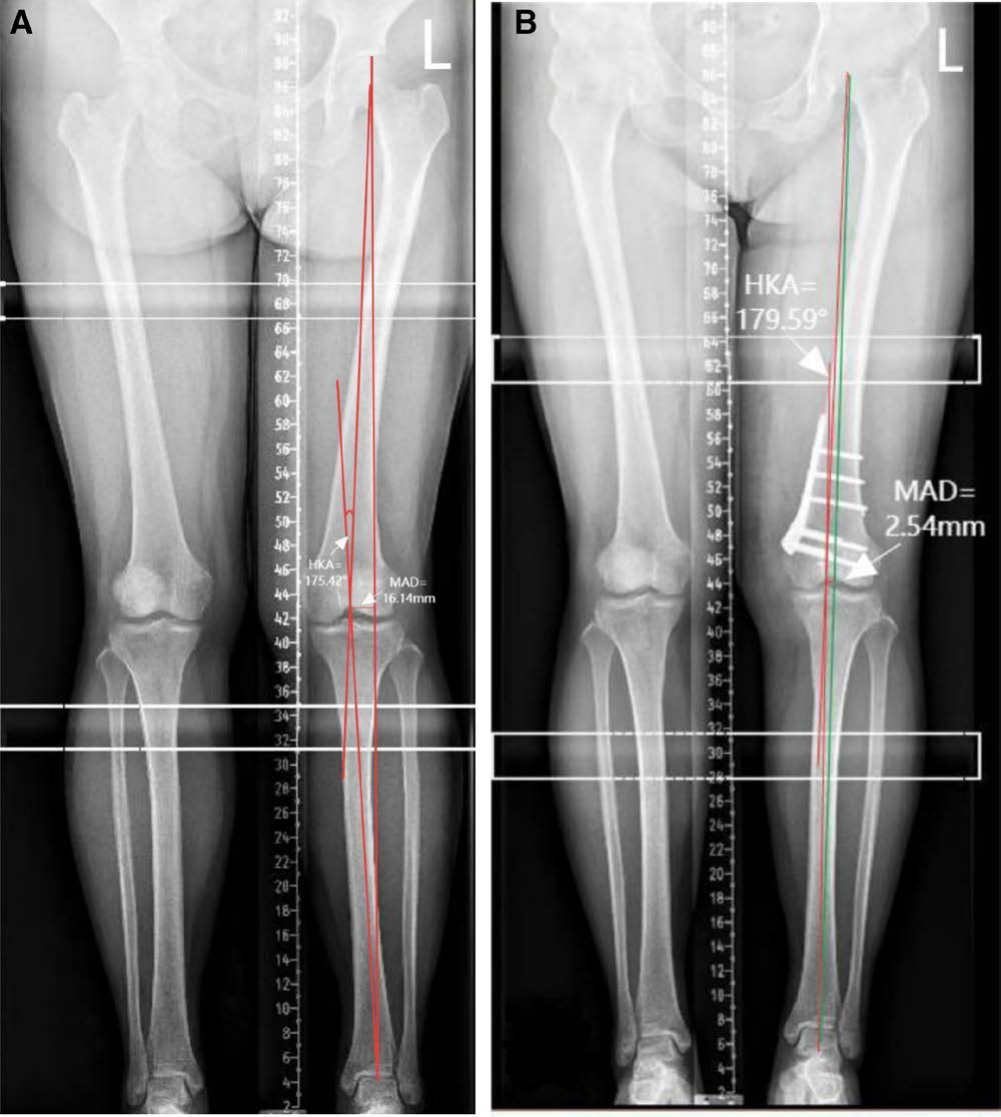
(A–D) Preoperative axial (A and B) and lateral (C and D) radiographs of the left knee demonstrating patellofemoral malalignment and significant lateral patellar displacement.
